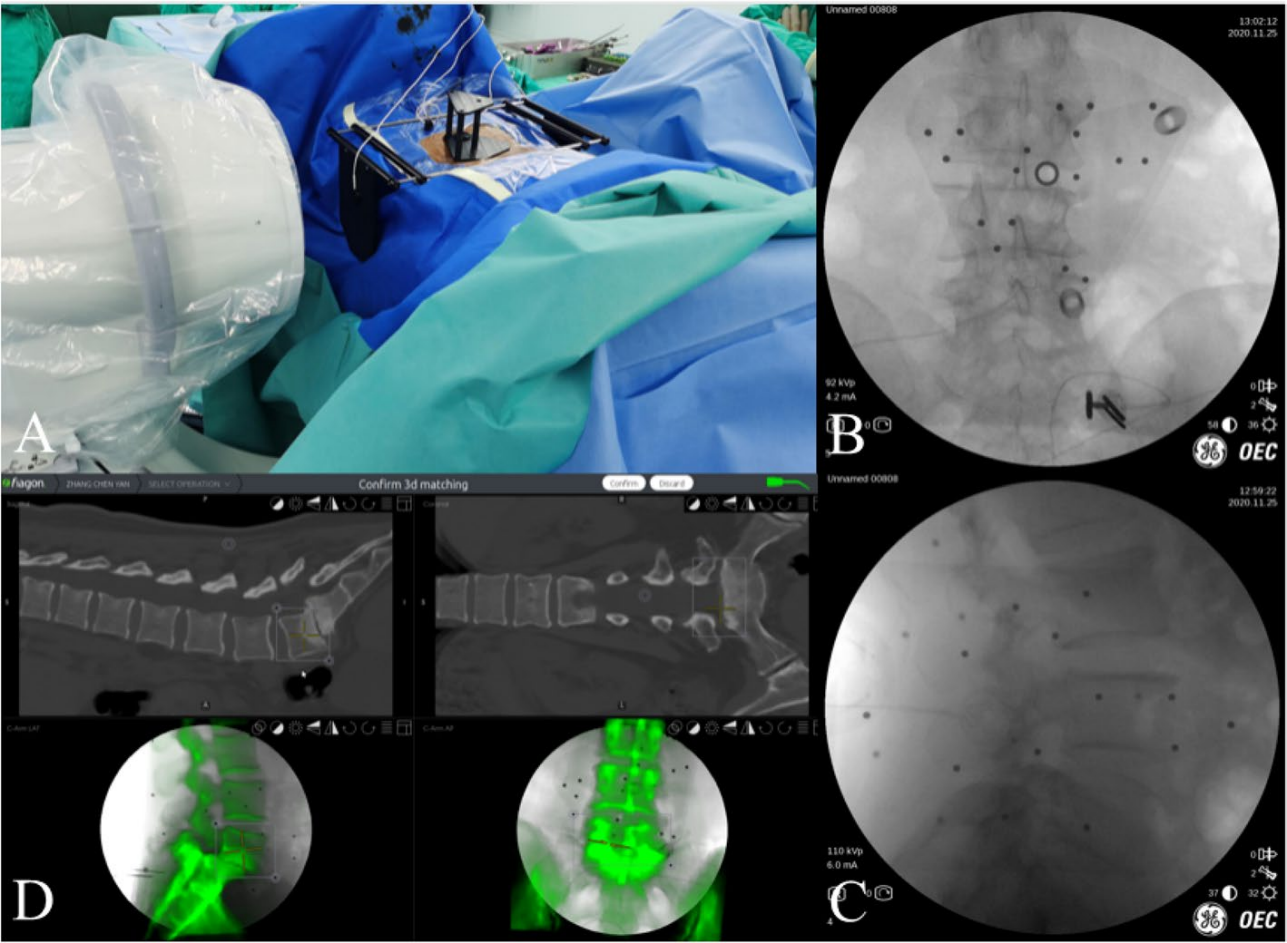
Preparation of Electromagnetic navigation. A After the field generator and a patient tracker equipped with signal coils was fixed on the ilium. B and C Anteroposterior and lateral fluoroscopic views of the lumbar segment were taken. D The software made surface matching on the respective vertebral body with the preoperative CT data in the electromagnetic coordinate system
